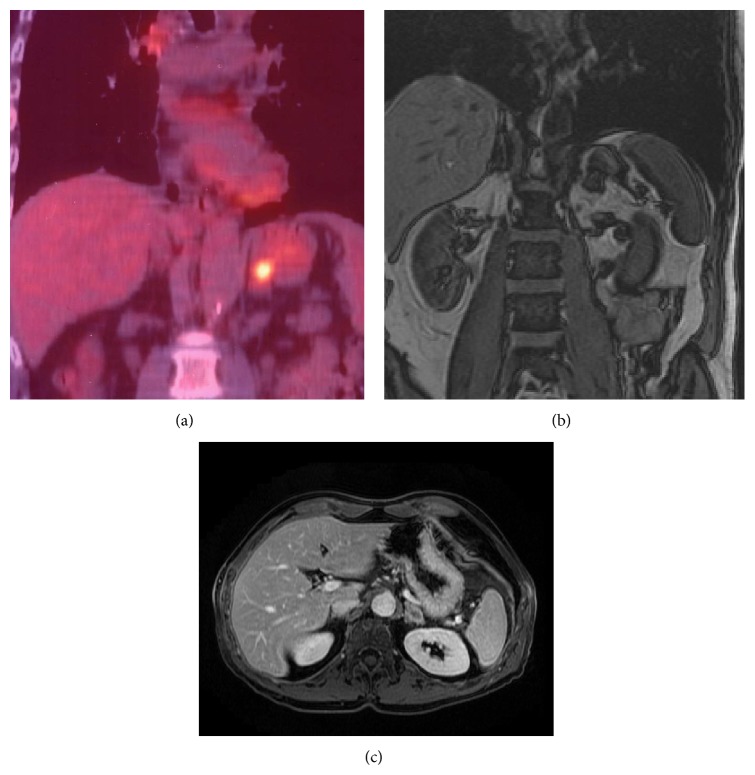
Images for a 70-year-old man with NSCLC (adenocarcinoma). (a) PET/CT showed intense ^18^F-FDG uptake by an adrenal metastasis. (b) Coronal out-of-phase image and (c) axial MR image with contrast medium confirming adrenal metastasis.
